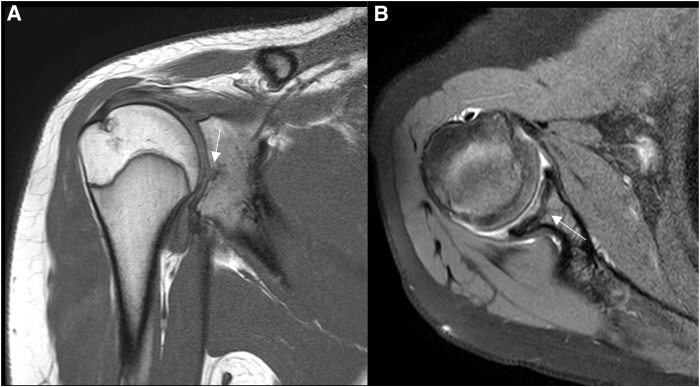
Magnetic resonance imaging of the right shoulder presurgery. An osteochondral lesion in the center of the glenoid is present. The left side shows a sagittal view in PDW-sequence, and the right side presents a transversal view in T1-sequence. The osteochondral lesion of the glenoid is demonstrated (→). It is centrally located in the glenoid.
